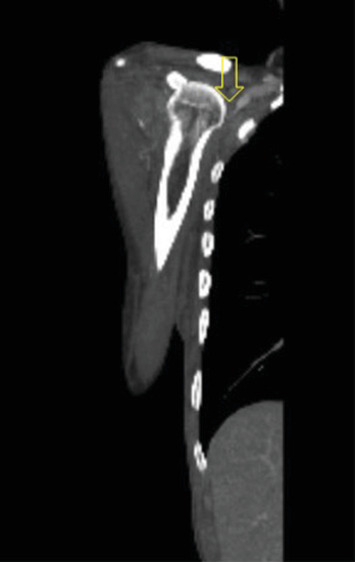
Contrast-enhanced CTA of the right upper extremity; the yellow arrow demonstrates compression of the axillary vein with absence of contrast opacification distal to the axilla.
